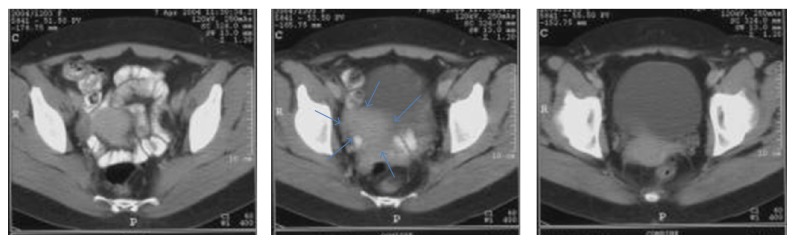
Computed tomography imaging of the pelvis (axial slices) demonstrating an abnormally enhancing right-sided pelvic mass (arrows).
